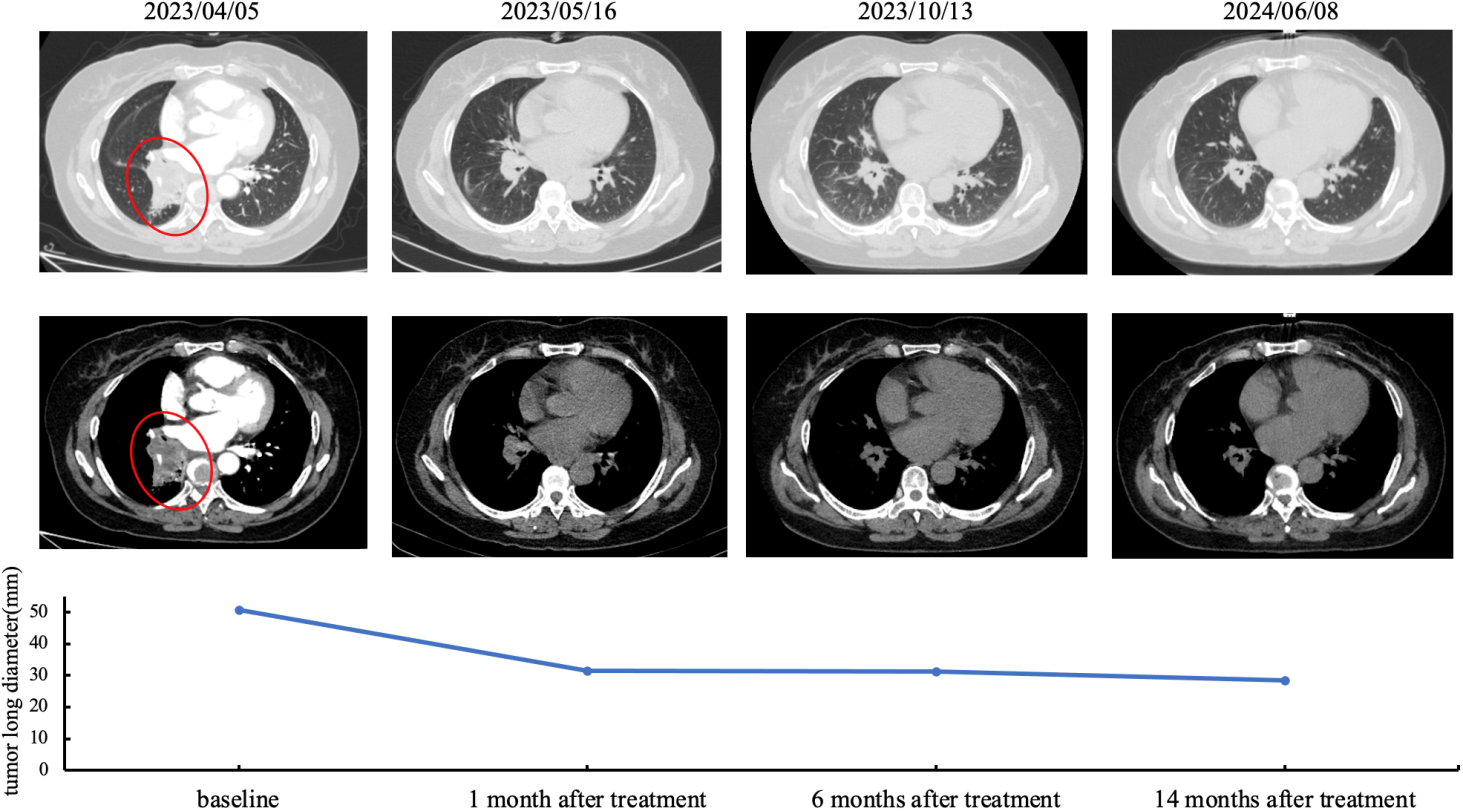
Treatment response was evaluated by chest CT imaging after lorlatinib. Representative CT images obtained with the lung window (upper row) and mediastinal window (lower row). Red circle indicates the location of the tumor on the lower lobe of the right lung. Line chart demonstrate the change of tumor long diameter (mm).
